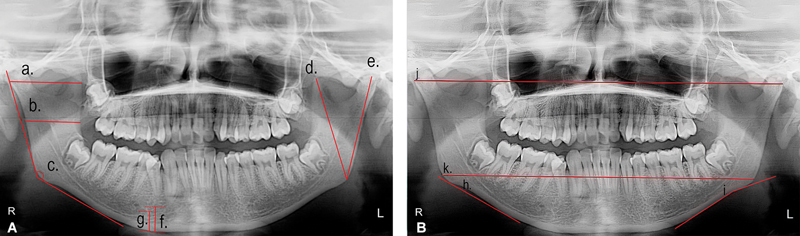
A representative orthopantomogram showing various anatomical landmarks: (
